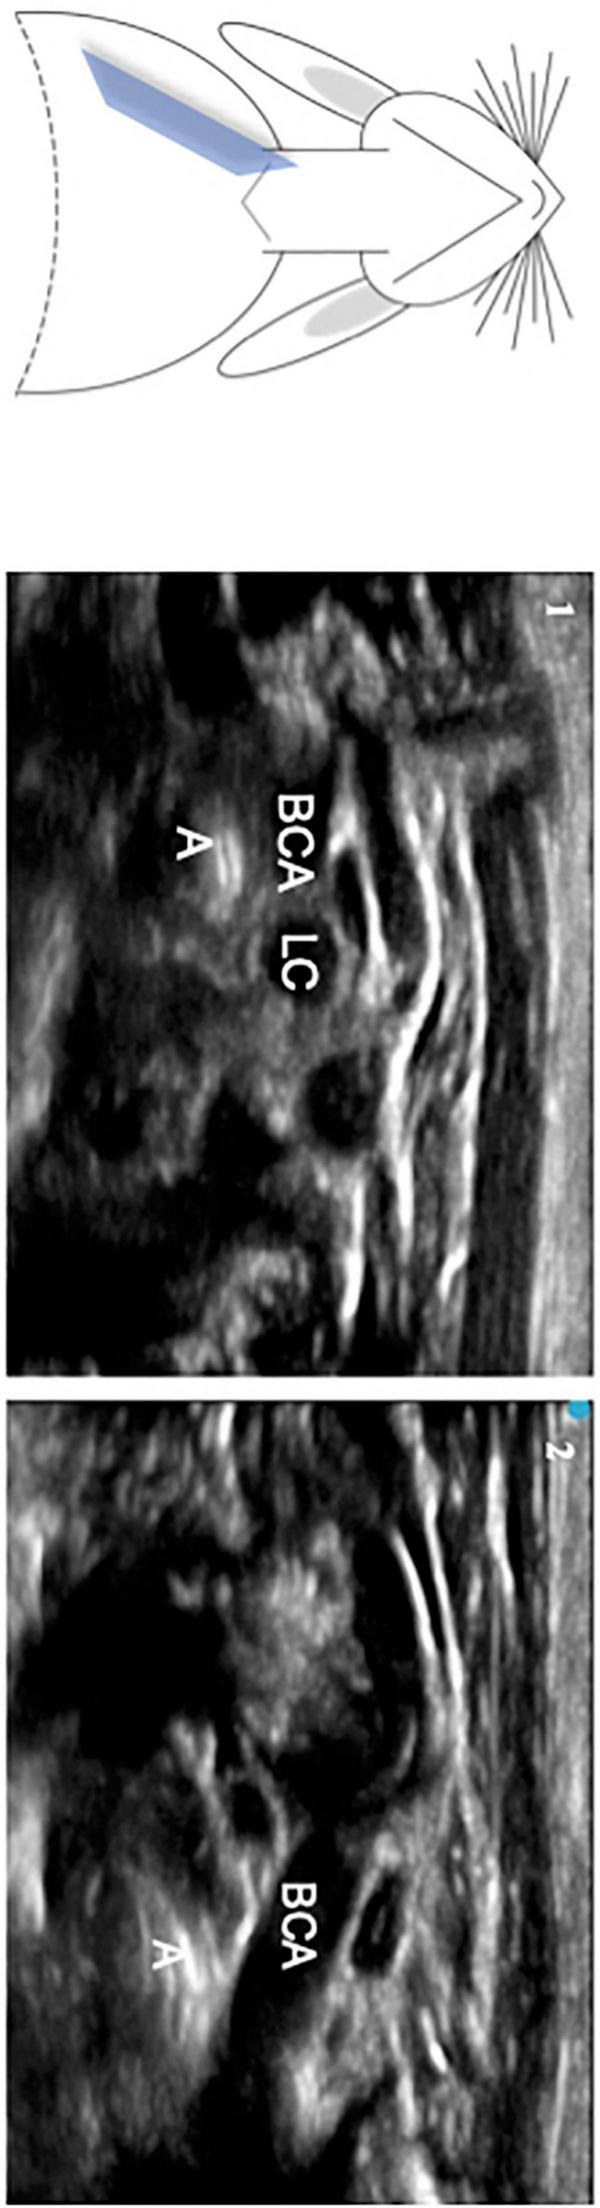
Oblique, parasternal, and midclavicular plane of the thoracic trachea. A – trachea, LC, Left common carotid artery, BCA, brachiocephalic or innominate artery. Part 1 – more cephalic view. Part 2 – more caudal view, at the level of the brachiocephalic artery.
